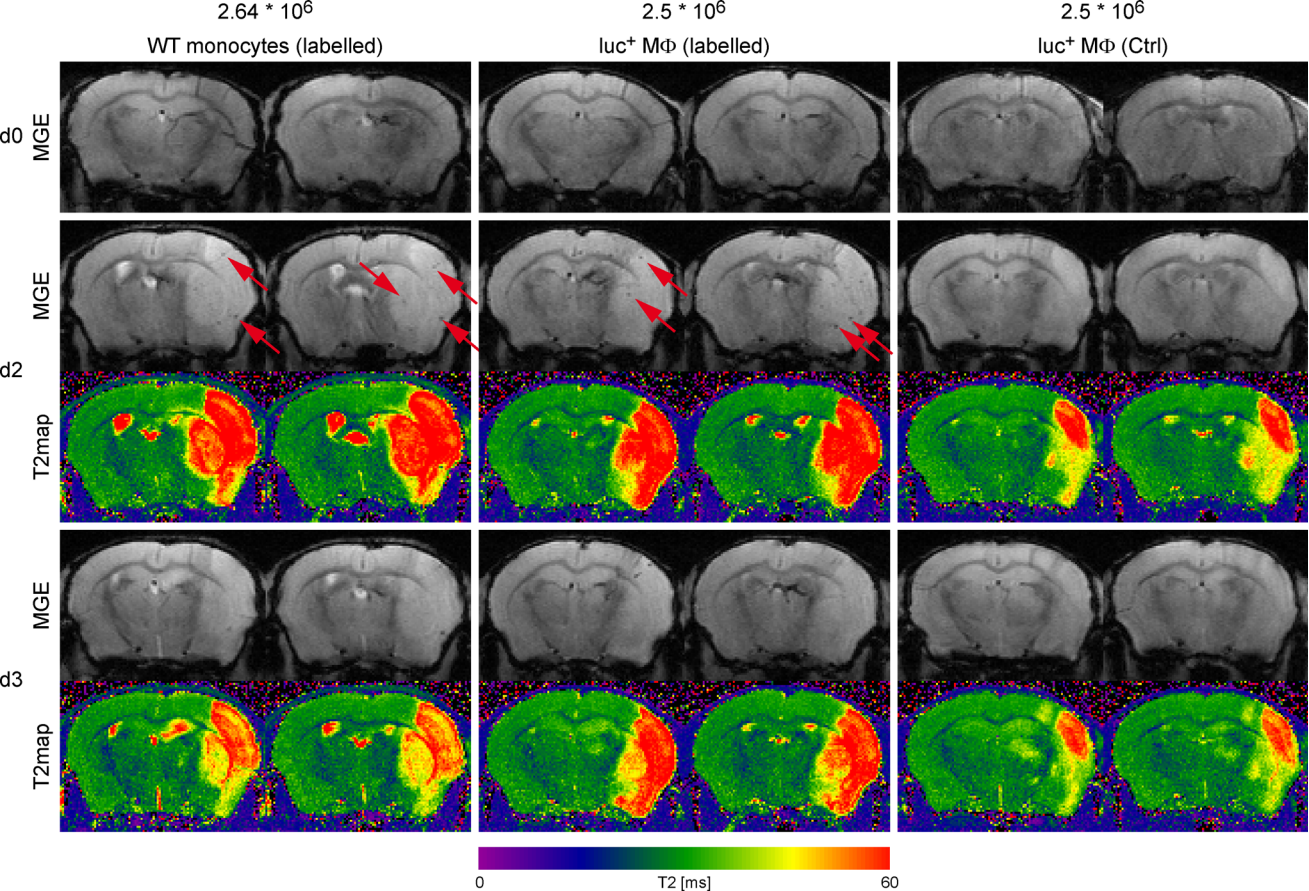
Representative MR images of i.v. injected WT monocytes and luc^+^ MΦ. Displayed are T2*-weighted images (second echo; TE = 8.5ms), acquired with a MGE sequence at 11.7T directly after MCAO surgery and before cell injection (upper row, d0), on day 2 as well as day 3 post stroke (1 and 2 days post cell injection) with corresponding T2 maps calculated for better stroke visualization. T2 relaxation times are displayed with the color scale bar below the images. Left column: contrast agent labelled WT monocytes (2.64x10^6^); center column: contrast agent labelled luc^+^ MΦ (2.5x10^6^); right column: unlabelled control luc^+^ MΦ (2.5x10^6^). Hypointense signal voids evoked by cells reaching the ischemic brain territory are indicated by red arrows.
